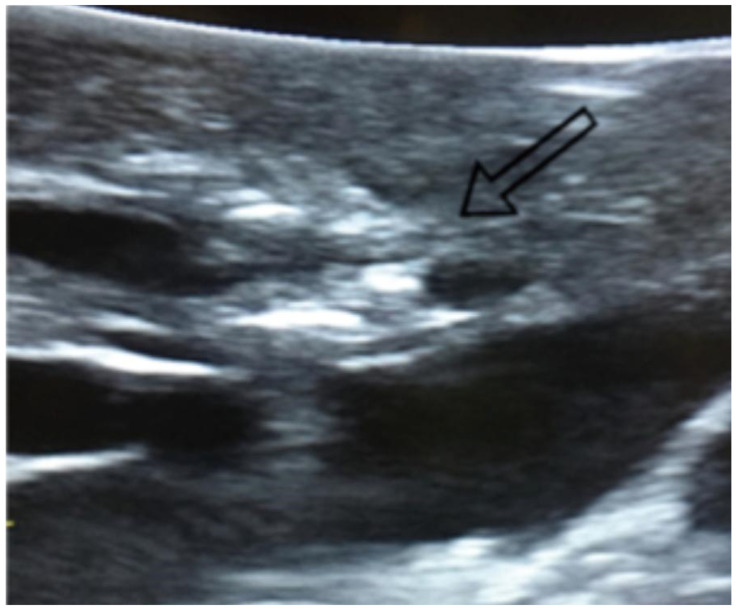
Visualization of lung nodules on intraoperative lung ultrasound. A pulmonary nodule is seen as a hyperechoic area, as indicated by the arrow.
